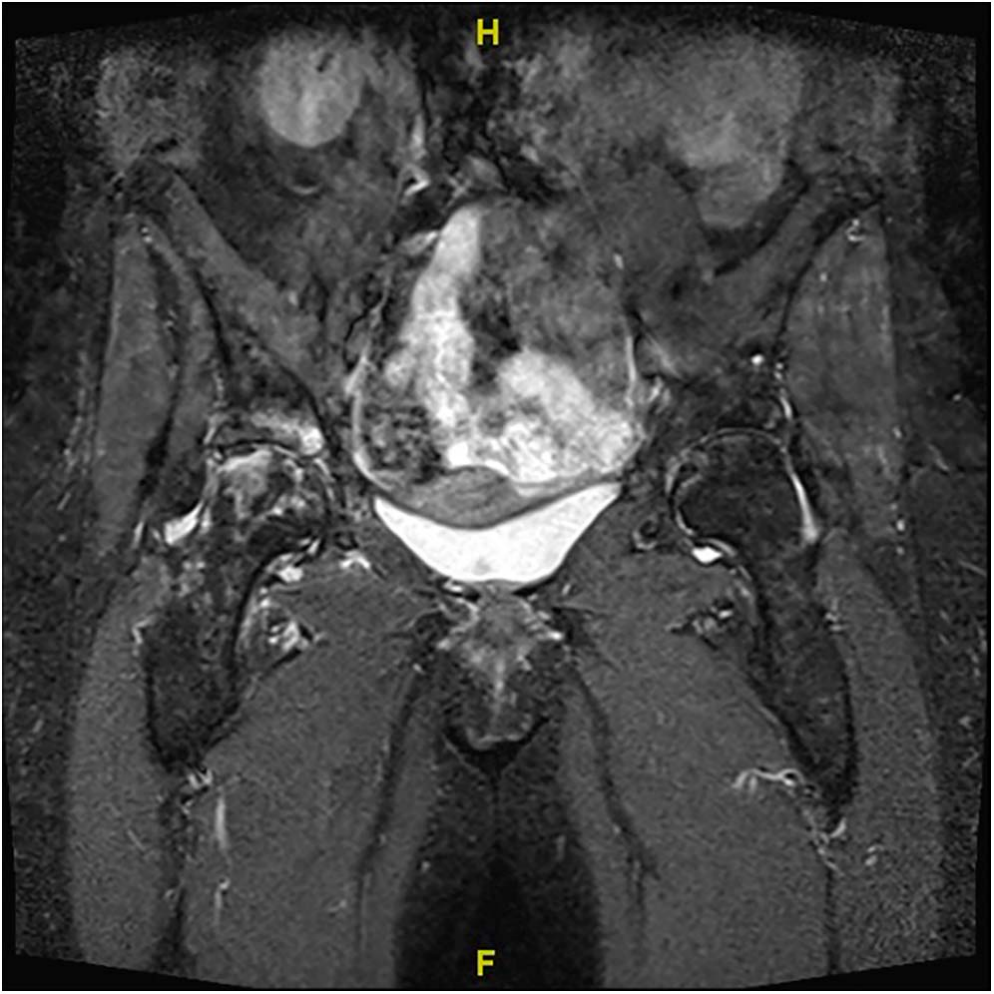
MRI image of bone and cartilage destruction of the right hip.
